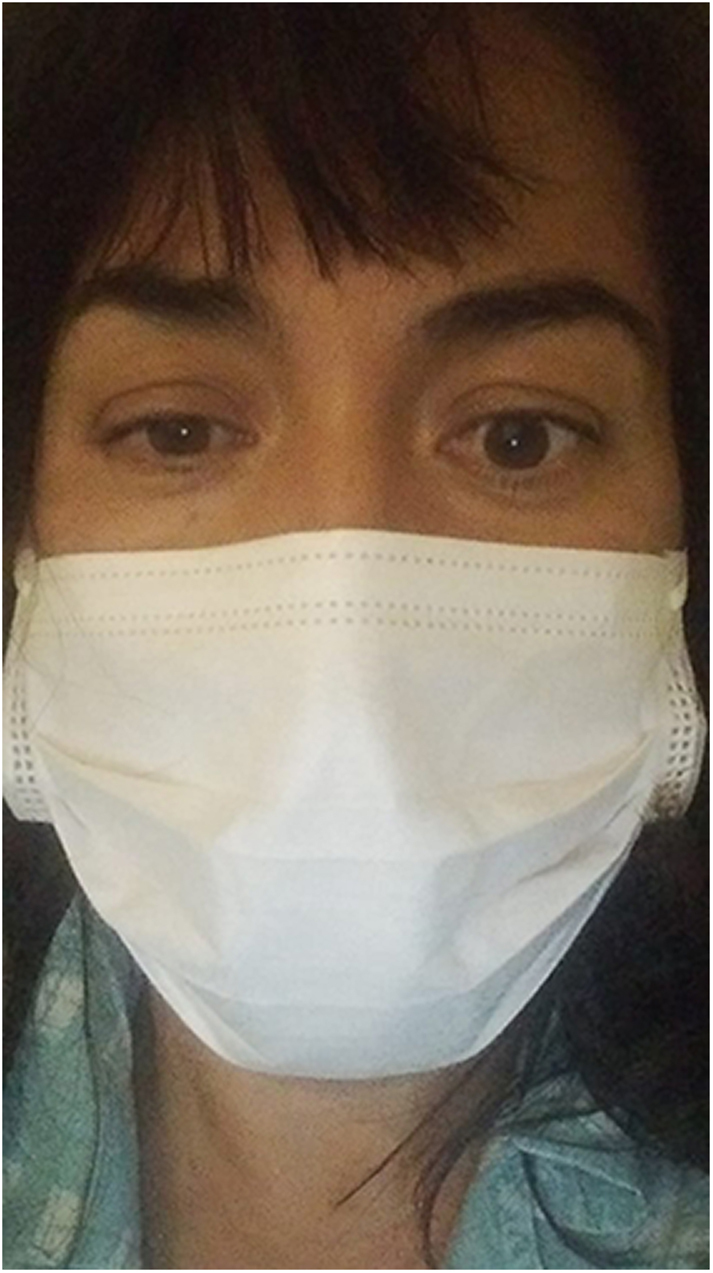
Anisocoria, miosis and partial ptosis of the right eye in a 48-year-old female patient after ultrasound-guided fine-needle aspiration puncture of a thyroid nodule located at the right lobe.
